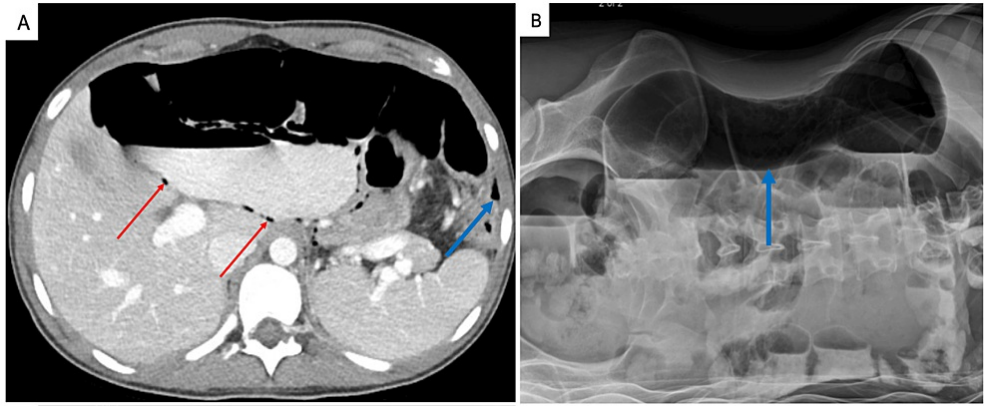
Computed tomography and abdominal radiograph demonstrating pneumoperitoneum and dilated loops of bowel A) Computed tomography axial image demonstrating multiple dilated loops of small bowel, intraperitoneal free air (blue arrow) near the left paracolic gutter concerning for perforated viscus, and pneumatosis intestinalis (red arrows) involving a small bowel loop.  B) Flat and upright abdominal radiograph demonstrating a large-volume pneumoperitoneum (blue arrow) and moderately dilated loops of the small bowel.
